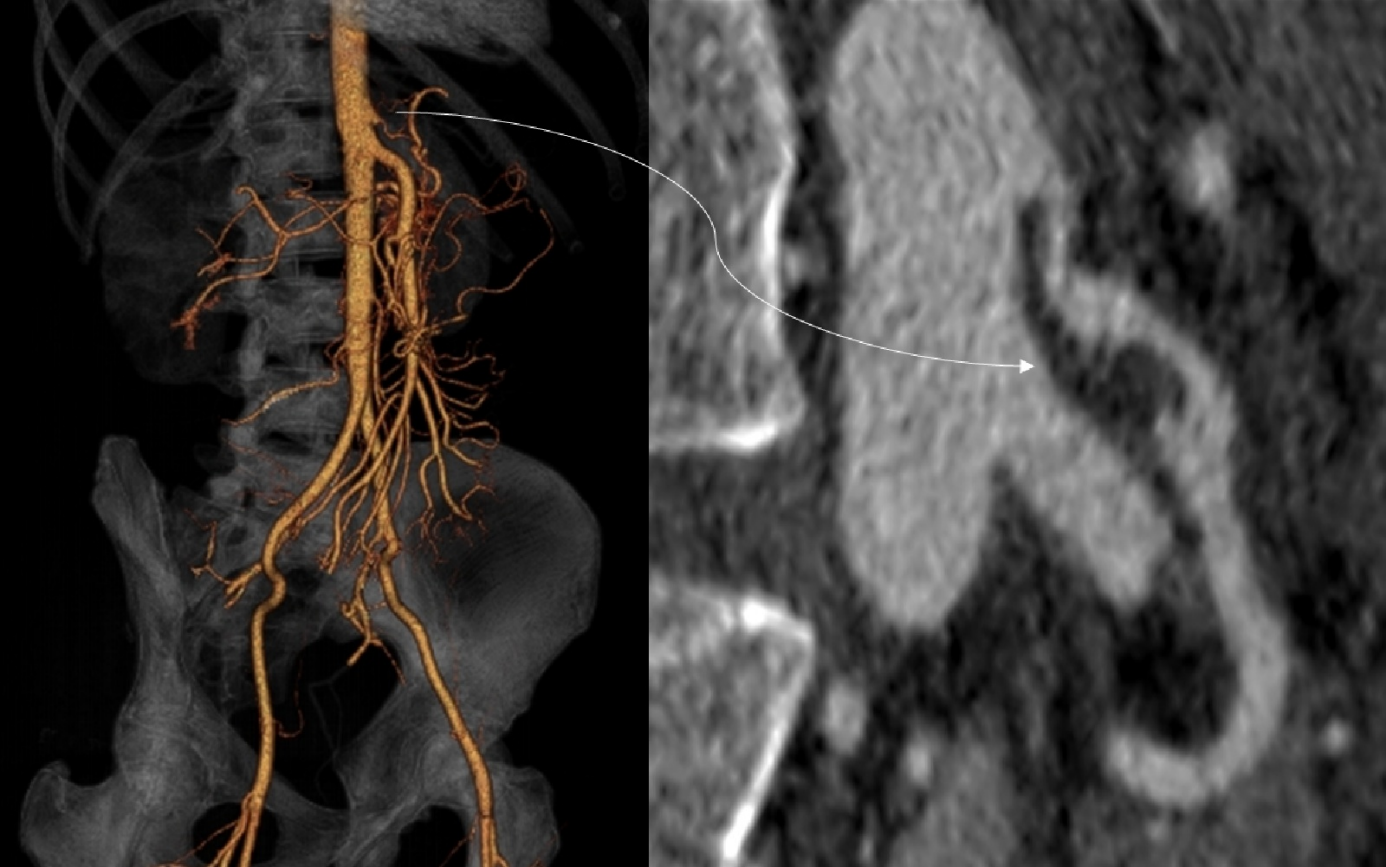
Volume-rendered and curviplanar reformatted computed tomography Images showing extension of the intramural hematoma into the celiac artery. This caused mild-moderate stenosis.
